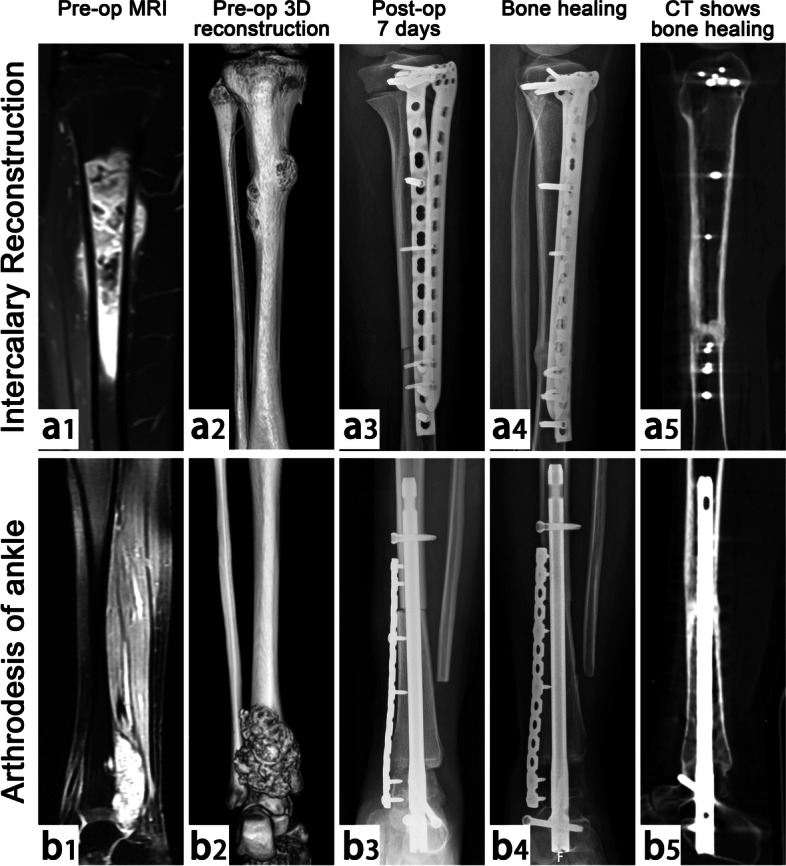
Limb salvage in 2 patients with stage IIB osteosarcoma of proximal tibia and distal tibia (case 2 and case 5). a1 and b1 Preoperative MRI examination and a2 and b2 CT 3D reconstruction graphics. a3 and b3 At postoperative 7 days, X-ray showed that the allograft matched well with the host and was firmly fixed. a4 and b4 At postoperative 16 months, X-ray showed that both junctions of the host–donor had healed, and no obvious loosening of internal fixation was found. a5 and b5 CT scan confirmed both junctions had healed completely
